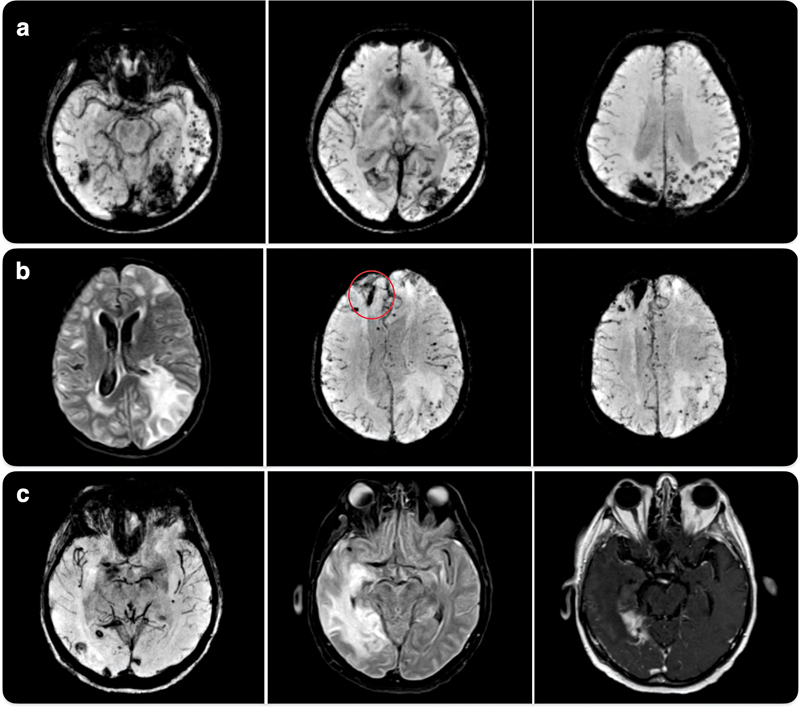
Brain magnetic resonance imaging (MRI) findings a. Case 1: Axial susceptibility-weighted imaging (SWI) MRI reveals multiple cortical and subcortical lobar microbleeds, predominantly in posterior regions, b. Case 2: Axial T2-weighted and SWI MRI demonstrate cortical and subcortical microbleeds, mainly in the left hemisphere, associated with asymmetric vasogenic edema c. Case 3: Axial SWI (left), fluid-attenuated inversion recovery (FLAIR) (center), and post-contrast T1-weighted (right) MRI show lobar microbleeds, cortical-subcortical vasogenic edema, and focal leptomeningeal enhancement, respectively.
